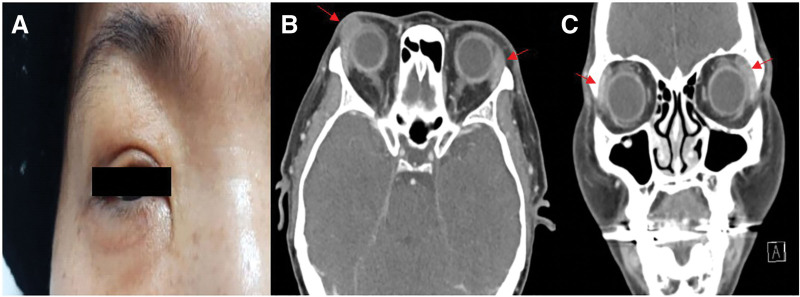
Clinical and radiologic findings at presentation. (A) External photograph of the right upper eyelid showing a painless mass without erythema. (B) Axial and (C) coronal CT images show diffuse, heterogeneous enlargement of both lacrimal glands, more prominent on the right. CT = computed tomography.
